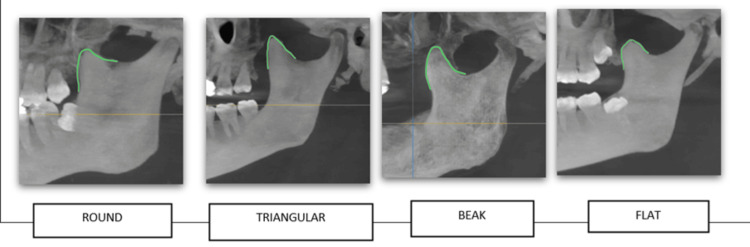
Tracing of morphological variation of the coronoid process in CBCT images CBCT: cone beam computed tomography
